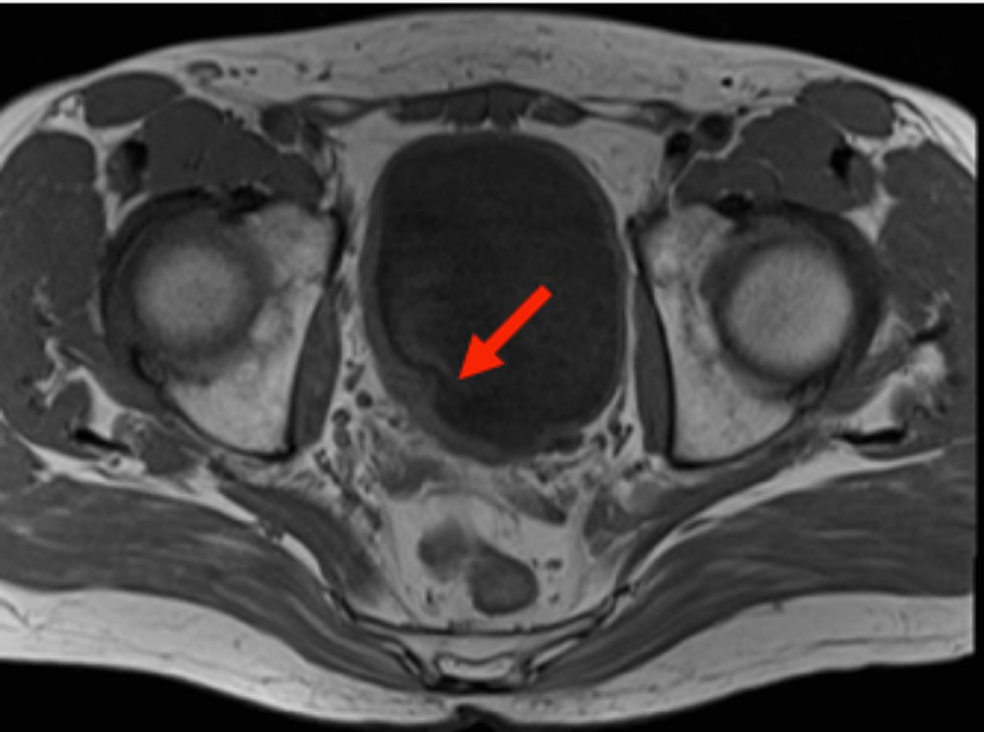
Pelvic MRI findings after the discontinuation of nivolumab. Pelvic MRI showing an irregular thickening of the right-sided wall of the bladder (red arrow). MRI = magnetic resonance imaging
